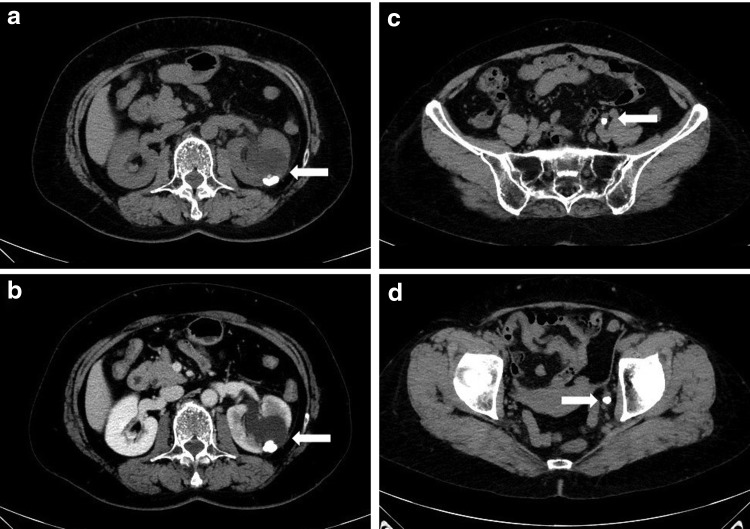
CT image at the time of visiting our hospital. (a) Plain CT image revealed a cystic lesion at the upper pole of the left kidney with apparent mural calcification posteriorly (*arrow*). (b) Contrast-enhanced CT image revealed that the low attenuation fluid within the cystic cavity when compared with the normally enhancing renal parenchyma confirms the presence of an upper pole caliceal diverticulum (*arrow*). (c, d) CT image revealed spherical calculi in the left lower ureter (U2–U3) (*arrow*).
